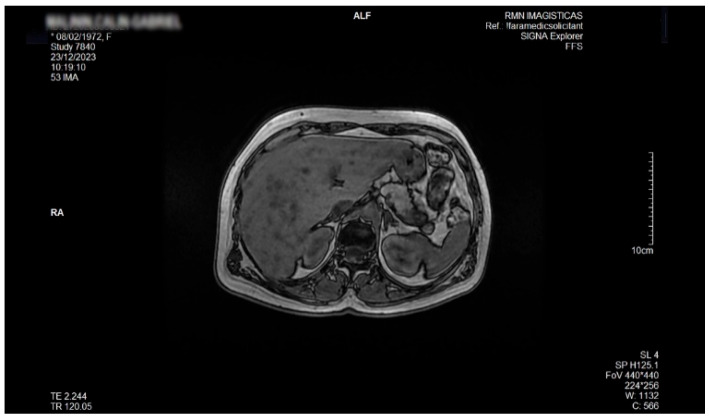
Axial out-of-phase MRI sequence demonstrating multiple disseminated focal hepatic lesions that exhibit marked signal drop, indicating intracellular lipid accumulation consistent with focal hepatic lipodystrophy.
